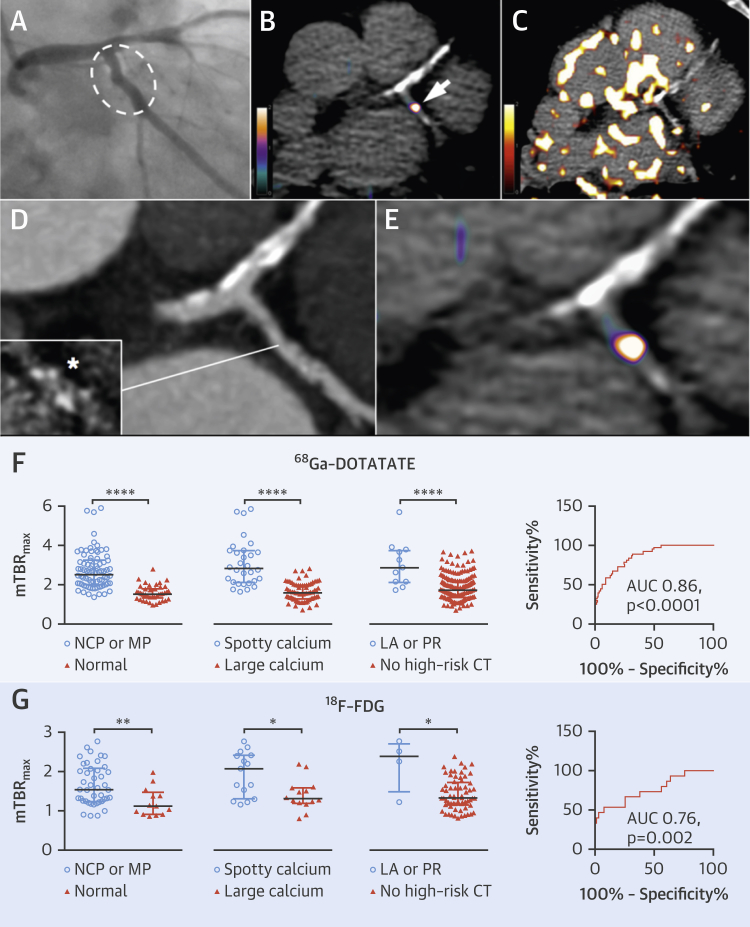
Coronary PET Inflammation Imaging: High-Risk CT Features (A) X-ray and (D) CT coronary angiograms of a 67-year-old man with stable angina, showing minor LCx atheroma (hatched oval) with spotty calcification ([inset] *calcium scan) and calcified plaque in the LAD artery. Although ^68^Ga-DOTATATE PET (B, E) allows unimpeded interpretation of inflammation in the LCx lesion (B, arrow), and lack of signal in the LAD, coronary [^18^F]FDG imaging is obscured by patchy myocardial tracer uptake (C). Graphs compare ^68^Ga-DOTATATE (F) with [^18^F]FDG (G) coronary TBR_max_ values by CT plaque morphology in coronary segments (^68^Ga-DOTATATE: NCP or MP, n = 86; normal, n = 45; spotty calcium, n = 30; large calcium, n = 72; LA or PR, n = 11; no high-risk CT, n = 186; [^18^F]FDG: NCP or MP, n = 43; normal, n = 13; spotty calcium, n = 15; large calcium n = 14; LA or PR, n = 4; no high-risk CT, n = 66), and ROC analysis demonstrating good diagnostic accuracy for high-risk coronary lesions. LA = low attenuation; LAD = left anterior descending; LCx = left circumflex; NCP = noncalcified plaque; MP = mixed plaque; PR = positive remodeling; other abbreviations in Figures 1, 2, and 3.
